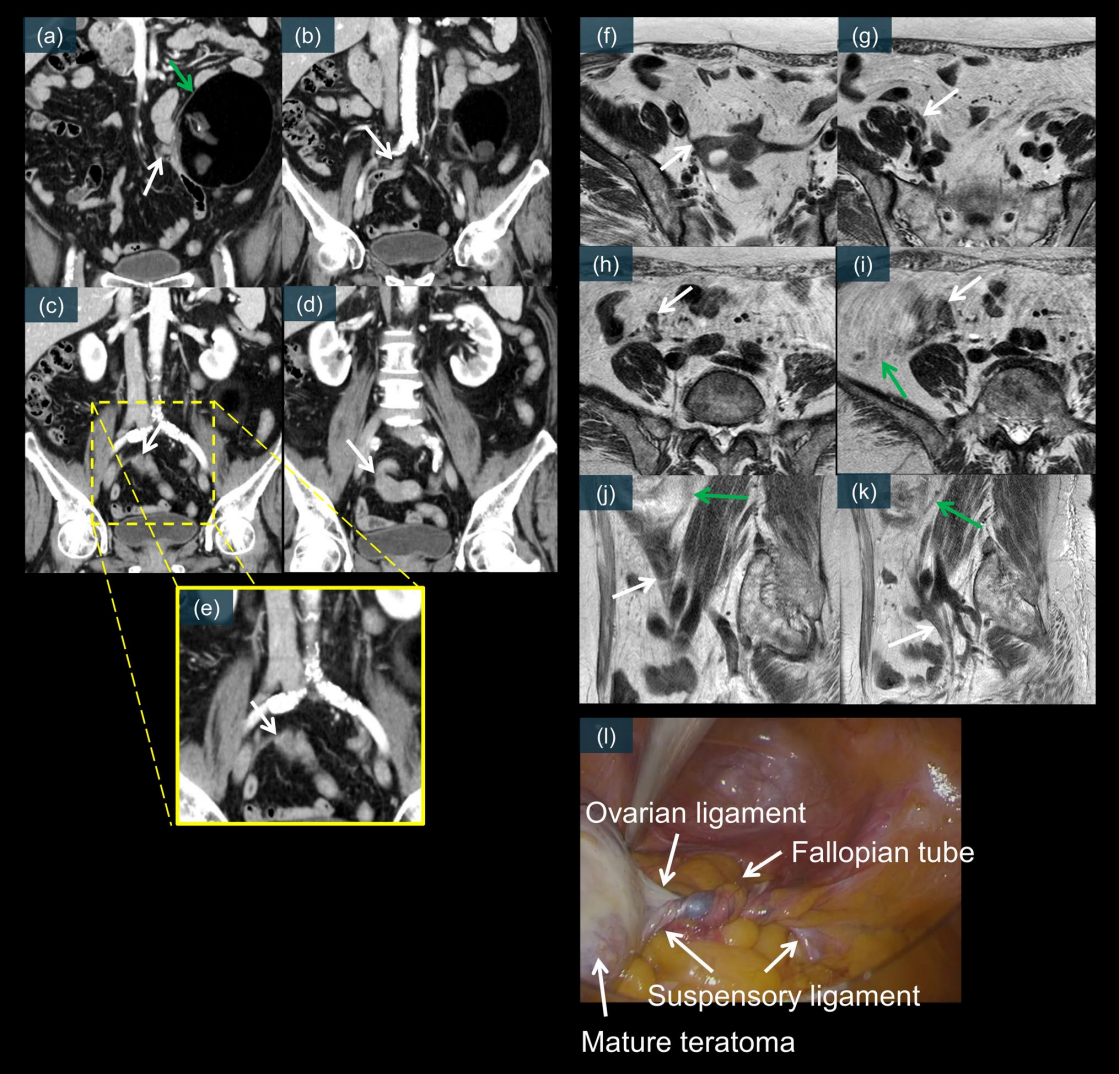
A 62-year-old female. a–d are contrast-enhanced CT images. The mature cystic teratoma is located in the left upper abdomen (green arrow). Although a clear whirlpool sign is not evident, a very long pedicle is observed continuing from the right uterine cornu (white arrows). A partially enhancing thin vascular structure is visible within the pedicle, suspected to be the ovarian artery (e, white arrow). f–k are axial and sagittal T2-weighted images. The mature cystic teratoma has moved to the right upper abdomen (green arrows), and the pedicle is depicted as a homogeneous low-signal-intensity structure (white arrows). No abnormal signals are observed on DWI or T1WI. l is a laparoscopic image, which reveals a 720° torsion of the right mature cystic teratoma and fallopian tube
